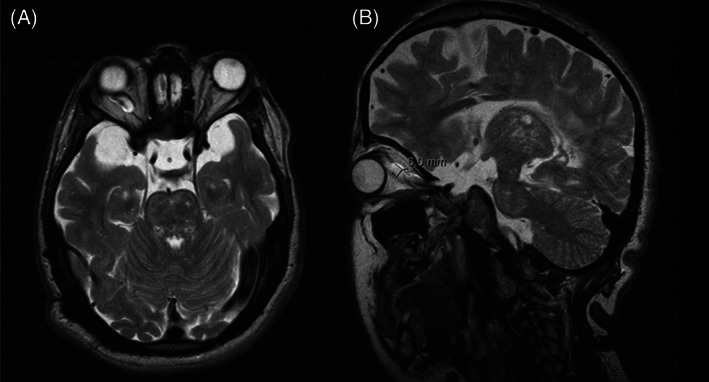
T2 ponderation MRI for patient 4 with evidence of enlargement of perioptic subarachnoid space. (A) Axial section. (B) Sagittal section
